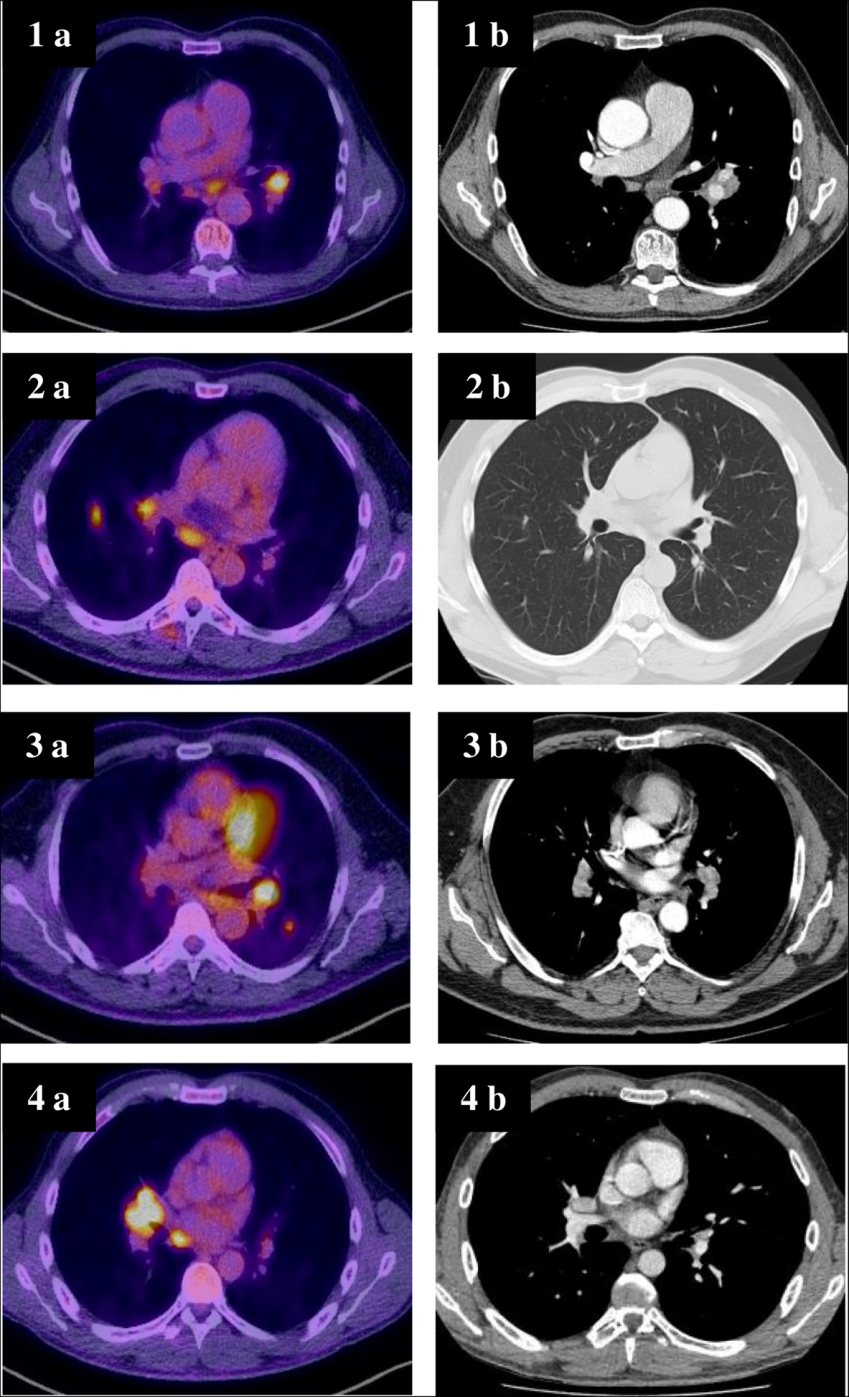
PET-CT and CT scans, respectively, of four patients suffering from pulmonary tularemia (initial presentation (a) and after completed antibiotic treatment (b)).
